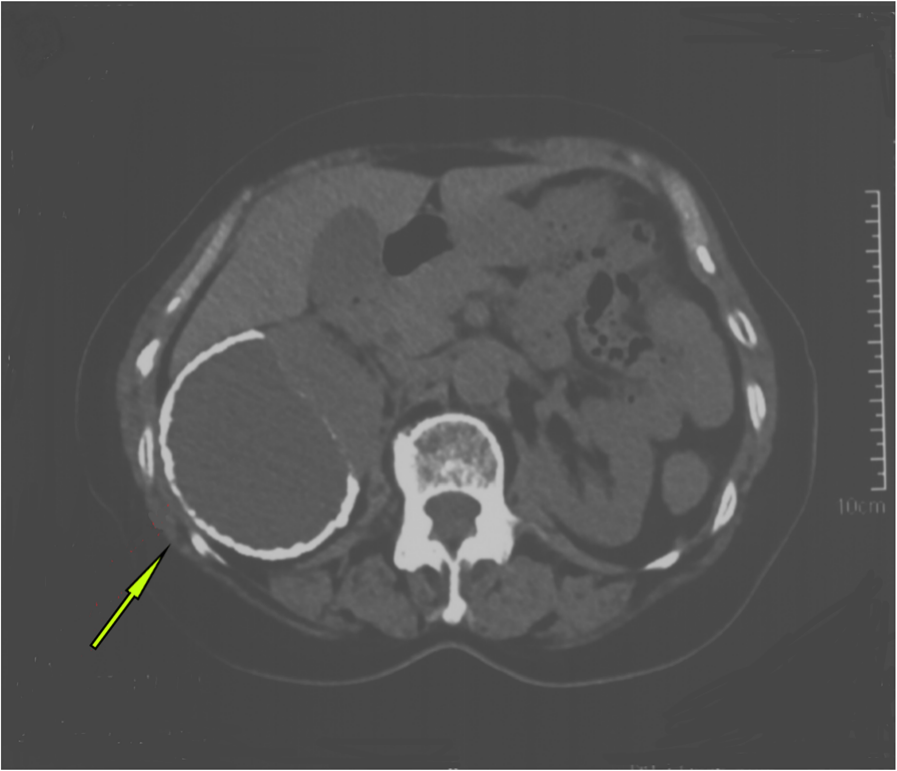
CT scan showing the annular calcification wall around the right kidney, with a size of 11.5 cm*6.9 cm*5.1 cm
